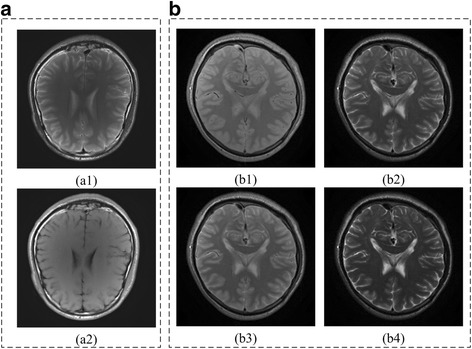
Multi-contrast brain MRI images. (A) are T2W and T1W images of one subject; (B) are images with 4 kinds of contrast for another subject by setting TE to different values
